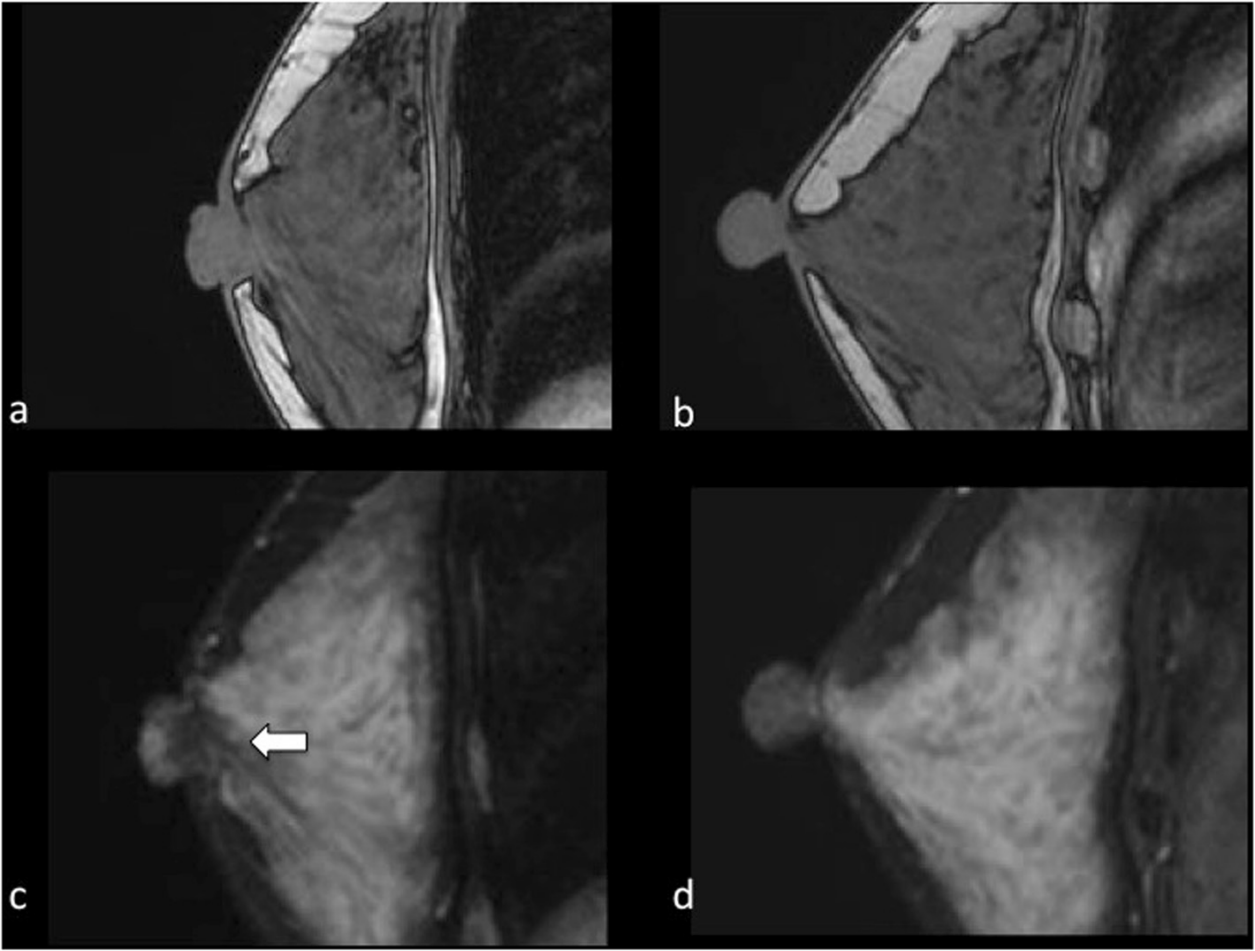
Magnetic resonance imaging. T1-weighted magnetic resonance imaging (MRI) did not show an obvious mass in the subareolar region of the right nipple (a). Conversely, T2-weighted MRI suggested a mass with low signal density (c
